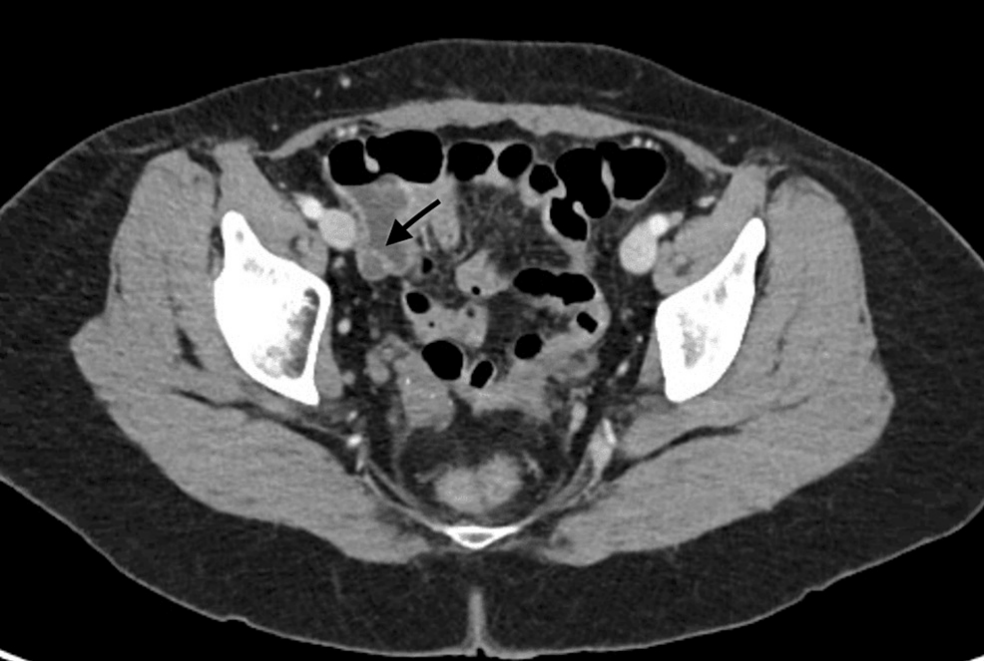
CT scan of the abdomen and pelvis without contrast showing an arrow pointing towards a mass in the Appendiceal region.
